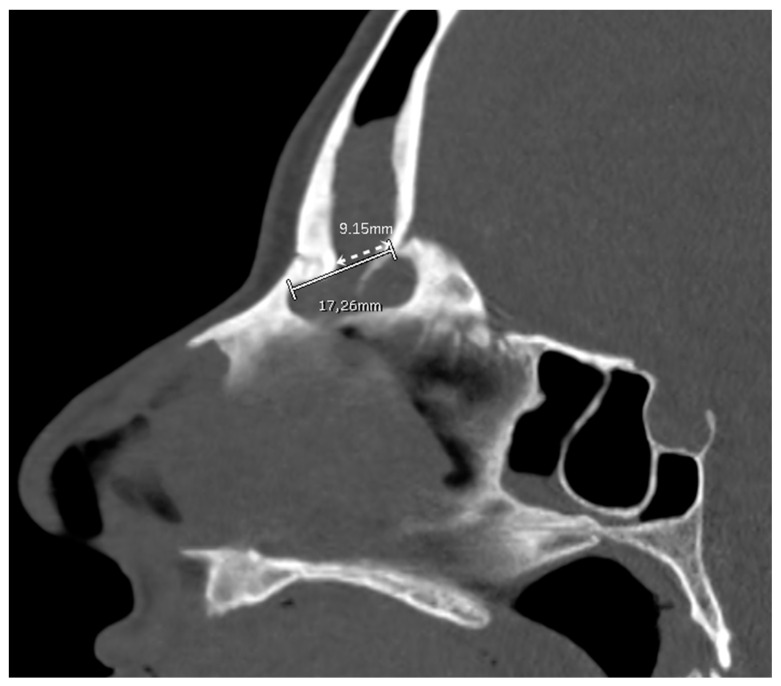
A patient undergoing a DRAF III for bilateral fronto-ethmoidal rhinosinusitis. On the pre-operative sagittal CT scan, the FOAP min (dashed white line with arrows) is measured as the distance between the posterior edge of the frontal beak and the posterior wall of the frontal sinus. The solid white line corresponds to the FOAP max.
